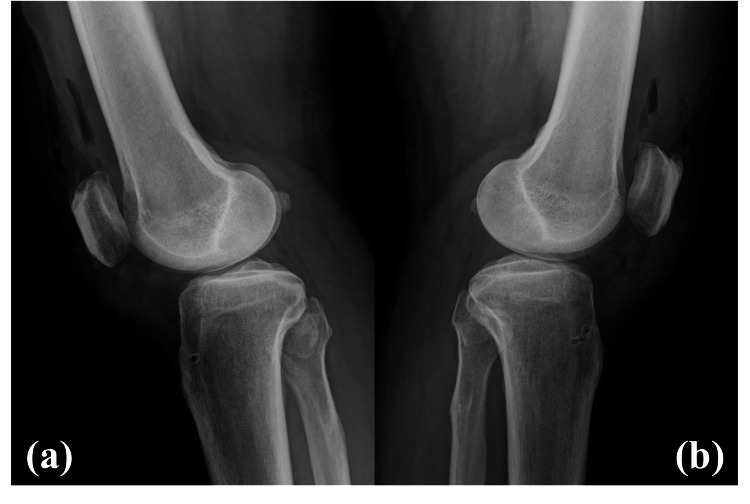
Postoperative lateral radiographs of the knees (a) right knee and (b) left knee. Both images demonstrate correction of patella alta after surgical repair.
